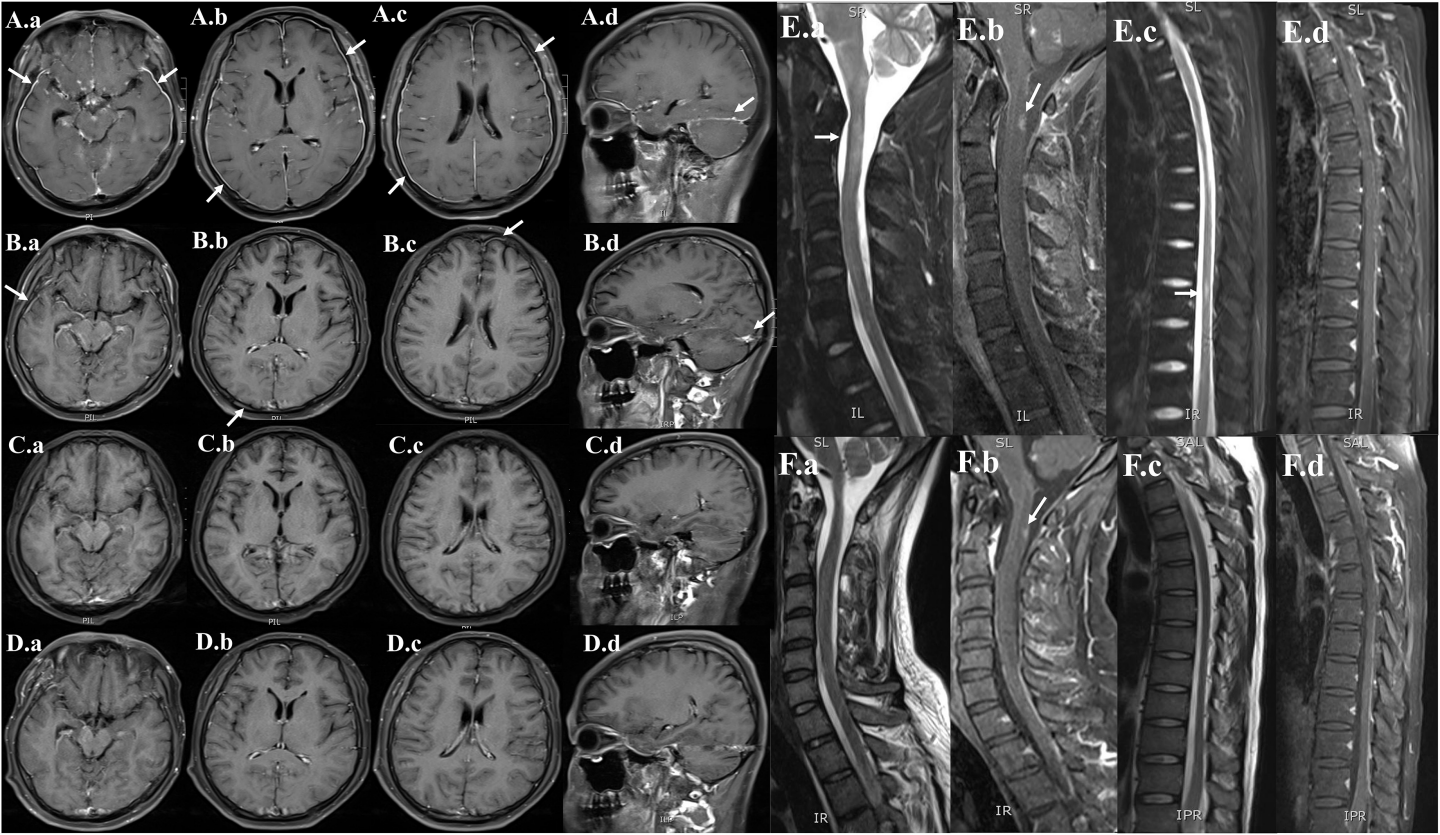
Serial neuroimaging with MRI demonstrating lesion amelioration. (A) Contrast-enhanced MRI brain (hospital day 5), (a,b,c) axial and (d) sagittal T1-weighted images showing diffuse leptomeningeal and pachymeningeal contrast enhancement (white arrows). (B) Repeat MRI on hospital day 29, (a,b,c) axial and (d) sagittal views showing less meningeal enhancement after 3 weeks of therapy. (C) Repeat MRI on the time of discharge (hospital day 62), (a,b,c) axial and (d) sagittal views showing the obvious resolution of the previous meningeal enhancement. (D) Repeat MRI on 6-month follow-up (a,b,c) axial and (d) sagittal views showing no meningeal enhancements. (E) MRI of the cervical and thoracic spine (hospital day 5), (a,c) sagittal T2-weighted and (b,d) post-contrast T1-weighted showing abnormal longitudinally extensive T2 weighted hyperintensities involving the C2–C3 and T6–T9. Partial enhancement was observed in the dorsal aspect of the spinal cord at C2 and T6–T7 levels (white arrows). (F) Repeat MRI on hospital day 29, (a,c) sagittal T2-weighted and (b,d) post-contrast T1-weighted images showing lesser abnormal hyperintensities compared with previously.
